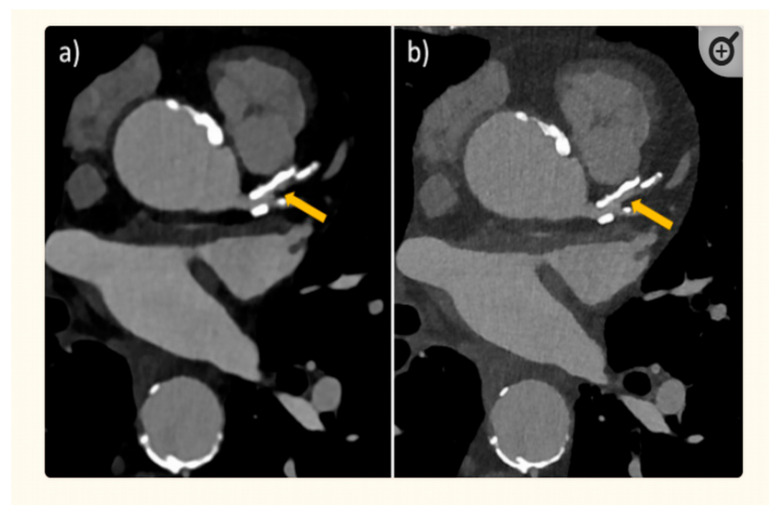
An 82-year-old man with coronary artery disease. The visualization of calcified plaques and the lumen diameter of the proximal left anterior descending coronary artery was improved via the acquisition of high-resolution photon-counting CT images (b) with 0.2 mm slice thickness rather than images obtained using standard CT (a) with 0.6 mm slice thickness. Arrow refers to larger perfused diameter of the proximal left anterior descending coronary artery in PCCT (b) than that observed with standard CT (a) which shows nearly occluded coronary lumen. Reprinted with permission under open access from Flohr et al. [24].
